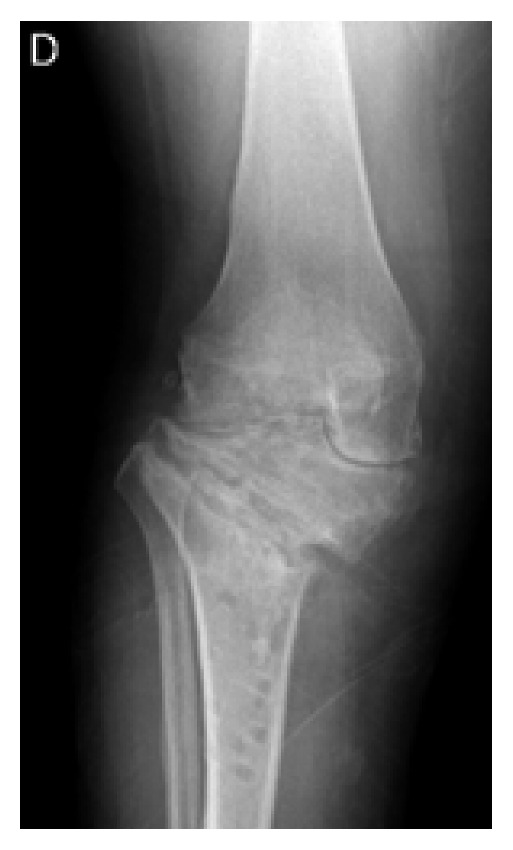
September 2013, after multiple debridements and arthroscopic washout and synovectomy, X-ray showing severe gonarthritis.
